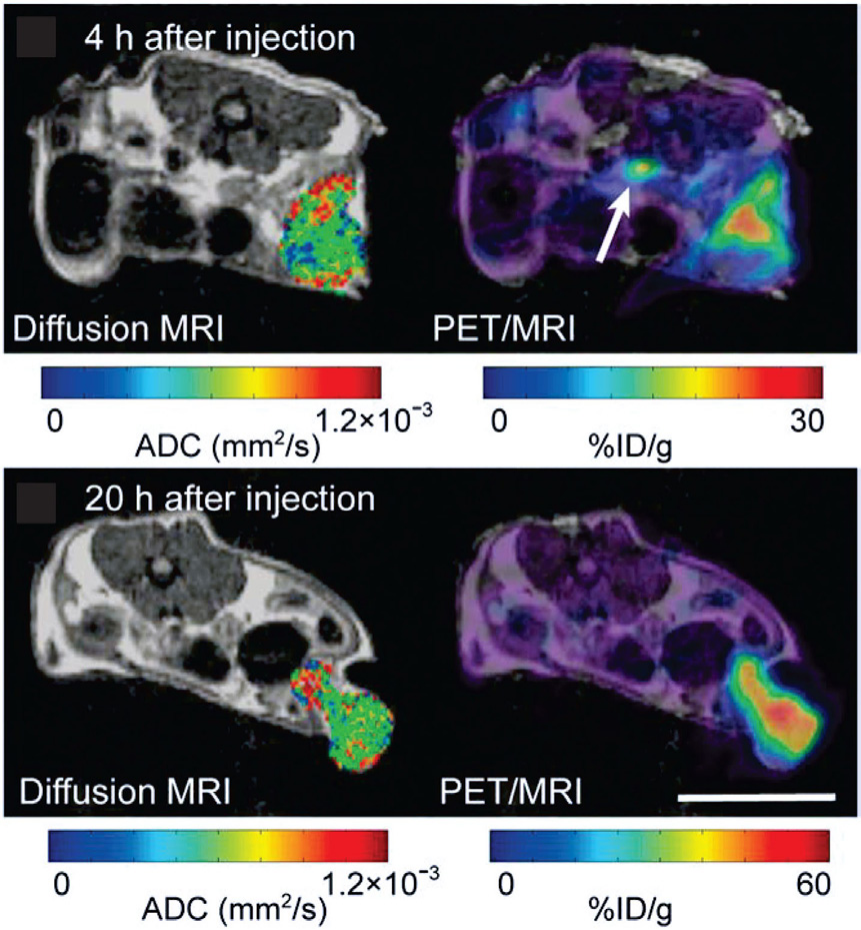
In a carcinoembryonic antigen (CEA)-expressing colorectal adenocarcinoma model, simultaneous magnetic resonance imaging (MRI)/positron emission tomography (PET) at 4 h and 20 h after the injection of a radiolabeled antibody against CEA (^64^Cu-DOTA-NHS-M5A) demonstrates its accumulation in the tumor over time, while the apparent diffusion coefficient across the tumor remained largely unchanged. Adapted with permission from Ref. (70) © by the Society of Nuclear Medicine and Molecular Imaging, Inc.
